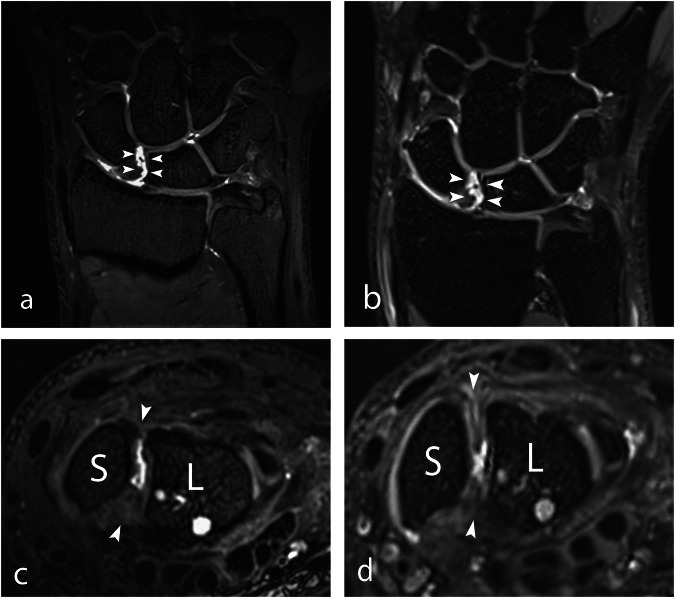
A 24-year-old male with right-sided wrist pain after a work-related injury. 3D PD-weighted MRI showed a clearly delineated SLL injury (arrowheads) on coronal and axial reformations at both (a, c) 7 T and (b, d) 3 T. SLL, scapholunate ligament; T, Tesla; S, scaphoid; L, lunate
